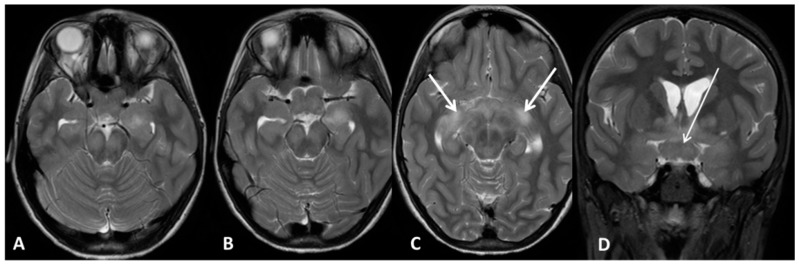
Optic pathways glioma 3B H+ (Subject 16 OPG). Brain MRI. (A,B,C) Axial T2 weighted images (w.i.); (D) coronal T2 w.i. with fat-suppression. All the panels show enlargement of the chiasm (arrow in D) and retrochiasmatic visual pathways with abnormal signal intensity (arrows in C). OPG: Optical pathways glioma; MRI: Magnetic resonance imaging.
